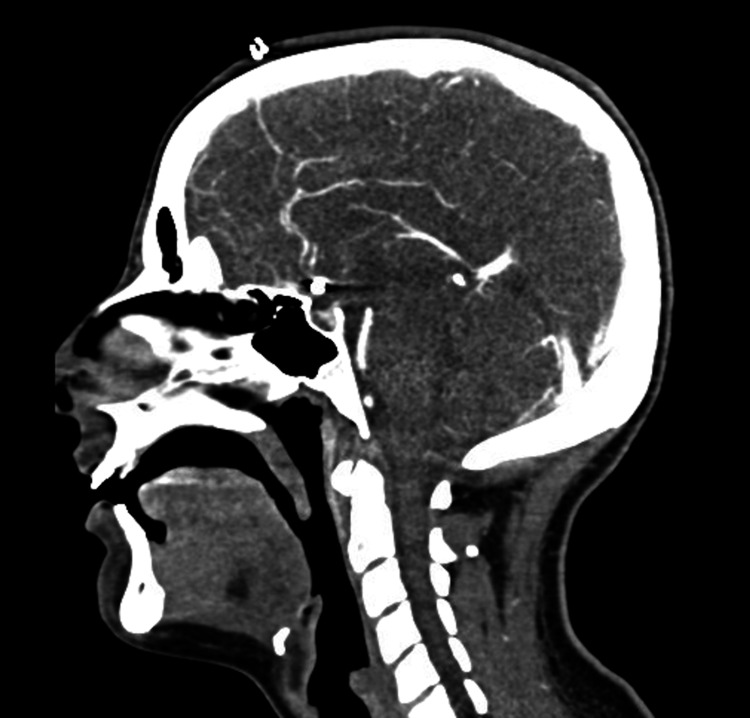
Computed tomography angiography post anterior communicating artery coiling
